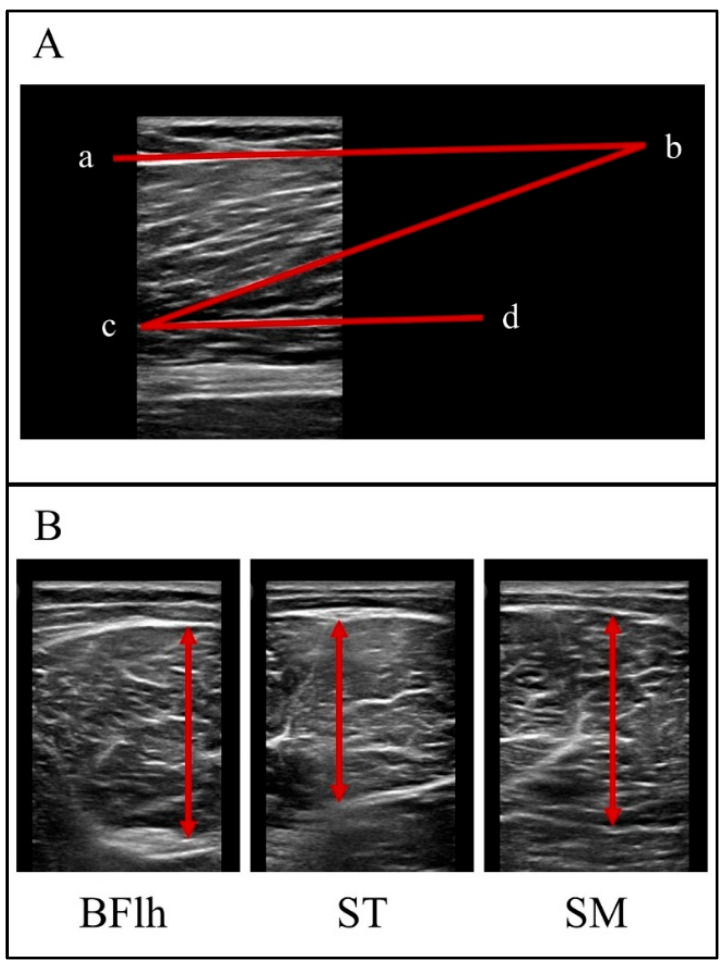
Analysis method for the ultrasonograms. (A) The BFlh FL was measured at 50% of the thigh length. Lines (ab) and (cd) indicate the positions of the superficial and intermediate aponeuroses, respectively. The line (bc) indicates the FL. (B) Muscle thickness of the BFlh, ST, and SM was measured at 50% of the thigh length. Arrows indicate the thickness of each muscle.
